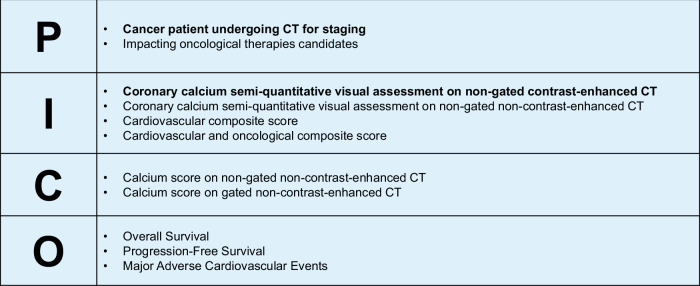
A theoretical PICO (Population, Intervention, Comparison, Outcome) framework addressing the role of semi-quantitative visual assessment of coronary calcium on non-gated contrast-enhanced CT scans in cancer patients
